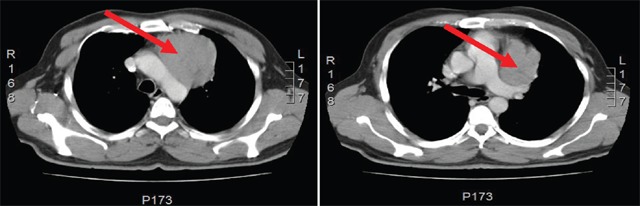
Computed Tomography of the chest shows the presence of an anterior mediastinal solid mass (red arrow) that has a maximum diameter of 7.5 cm. This tumor has a fine line of separation from adjacent vascular structure and back side of the sternum and rib cartilage.
